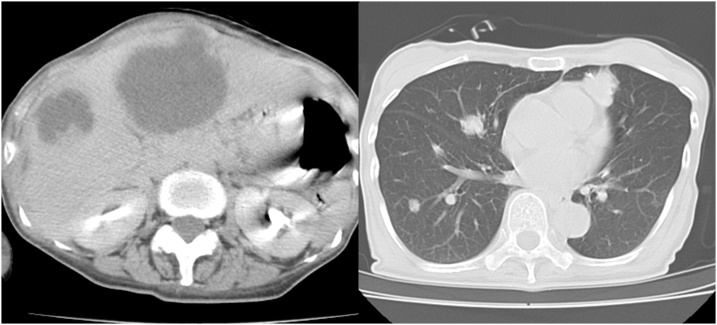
Abdominal contrast-enhanced CT findings 3 months after surgery Liver and lung metastases rapidly increased in size.
